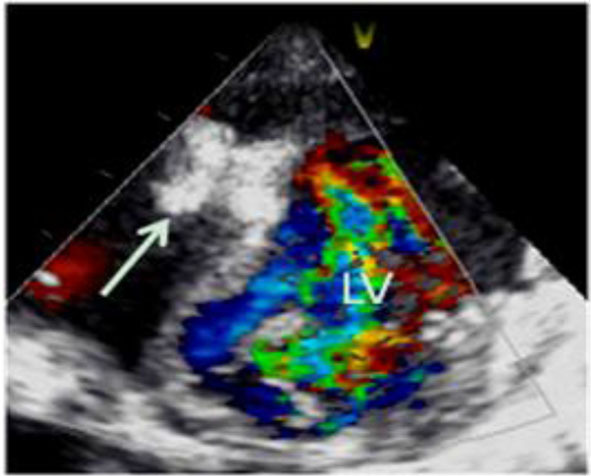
Transthoracic echocardiogram showed no residual shunt after operation, and the arrow indicates the occluding device.
